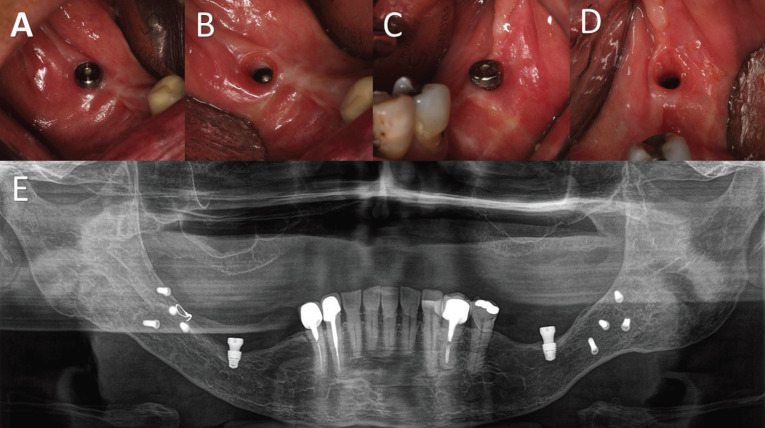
In the follow-up periods, if the healing screw were more than 1mm above the soft tissues, they were changed to a smaller one. A, C. Replacement of the healer on the right side and left side respectively. B, D. Soft tissue aspect without the healer bilaterally. E. Panoramic postoperative radiography from the same patient showing the Neodent WS short implant and healing screw. The screws in the mandibular angle are from the rigid internal fixation of a previous orthognathic surgery.
